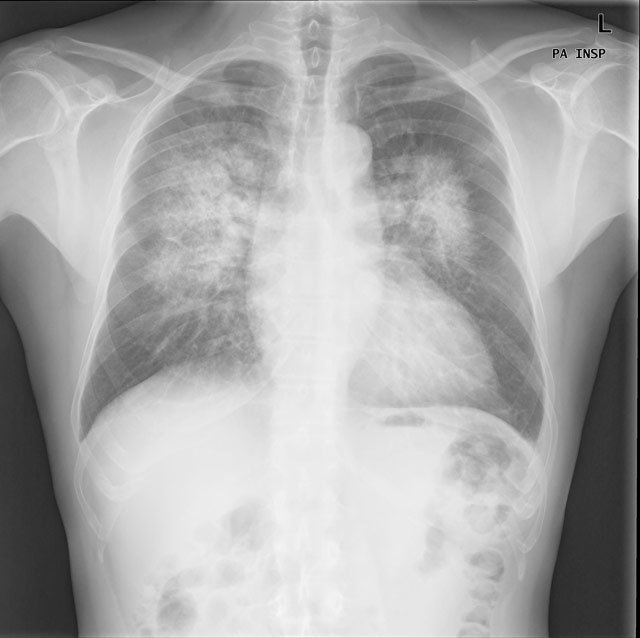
Chest radiograph (PA view) showing bilateral perihilar alveolar infiltrates. The heart size is not enlarged and there are no pleural effusions.
